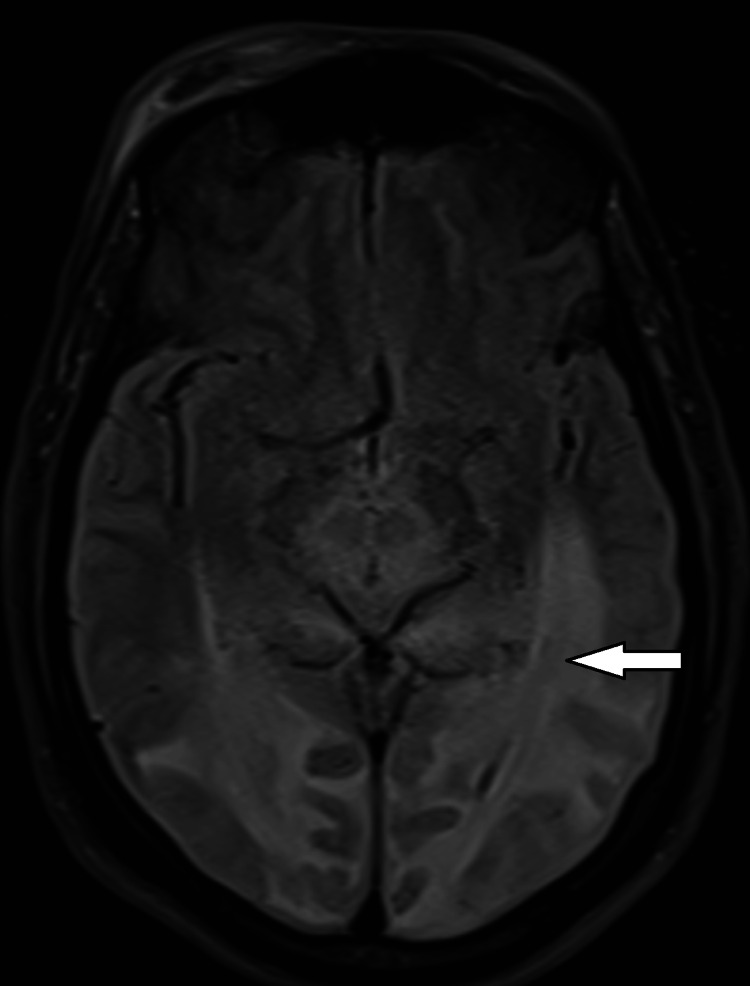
T2 FLAIR Sequence on Magnetic Resonance Imaging in Case 1 The white arrow indicates hyperintensities, representing vasogenic edema. FLAIR: Fluid-attenuated inversion recovery
